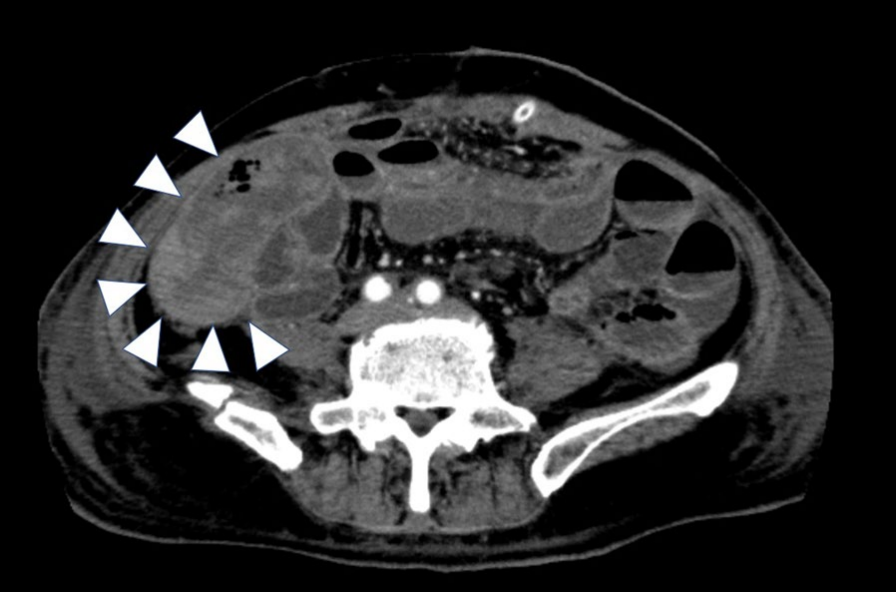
CT findings 10 days after surgery. CT showed wall thickening and a decrease in contrast effect in some small intestines (arrowhead)
